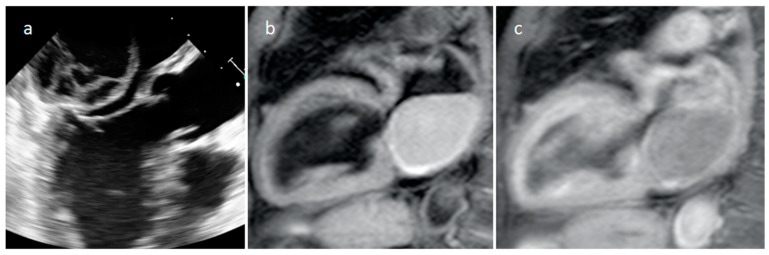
Echocardiography (a), T1-weighted (b) and T1-weighted gadolinium-enhanced MR sequences (c) in a patient with spontaneous acute left atrial hematoma.
